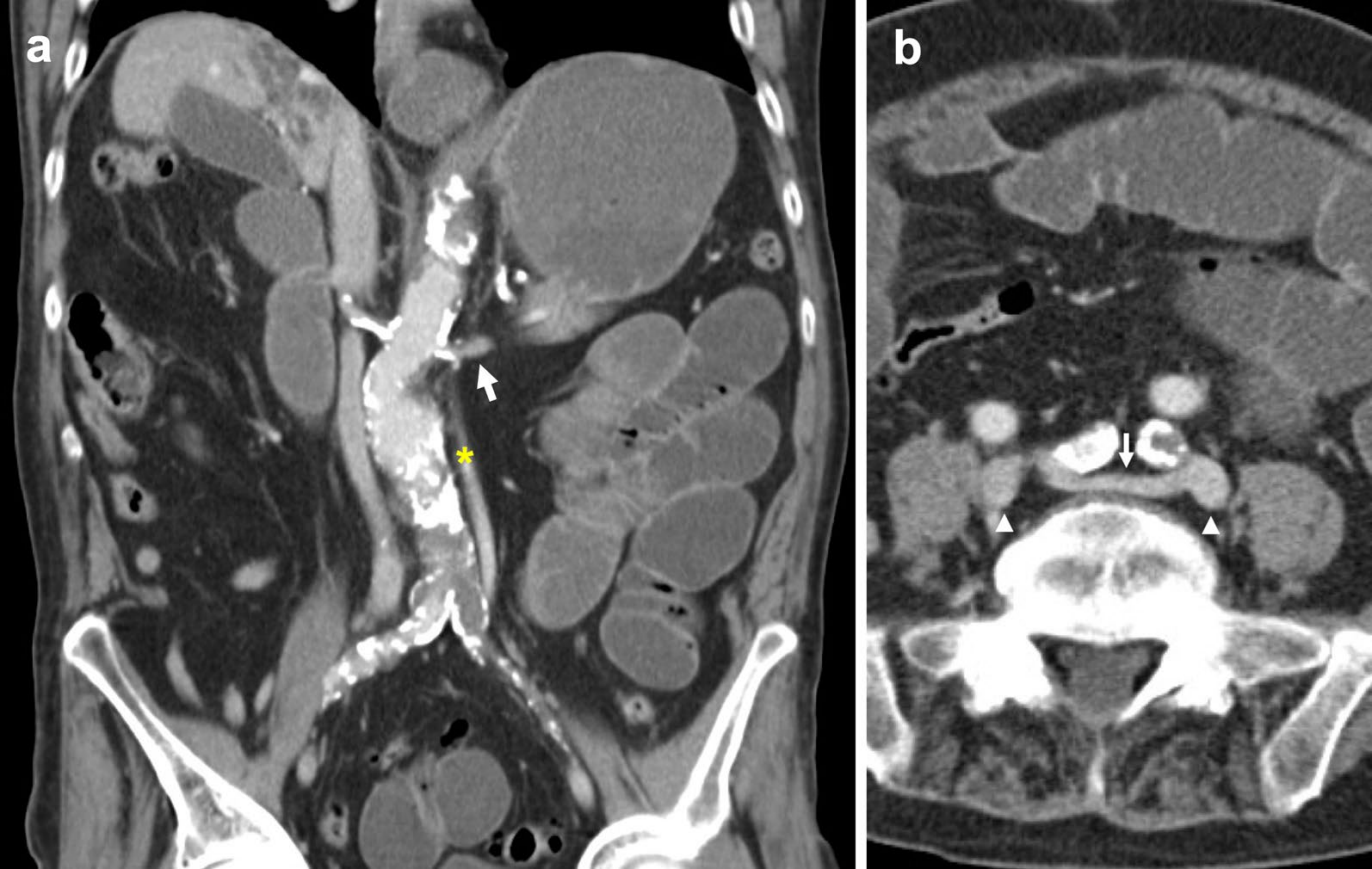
Duplicated IVC with asymmetrical caliber and bridging vein. a Contrasted-enhanced coronal CT shows a duplicated IVC (asterisk) of smaller caliber compared to the orthotopic IVC, that approaches and subsequently drains into the left renal vein (arrow). b Axial CT image shows a bridging vein (arrow) connecting paired common iliac veins (arrowheads) positioned anterior to the L4 vertebral body and posterior to common iliac arteries
